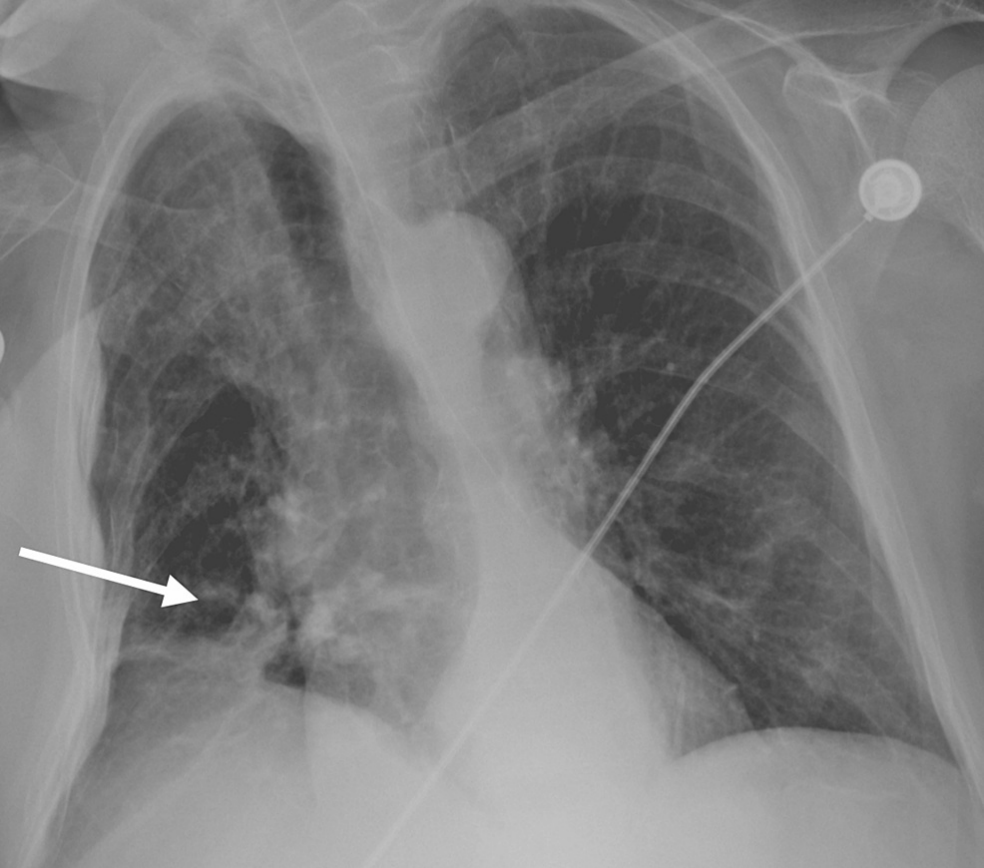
Chest x-ray documenting aspiration pneumonia
